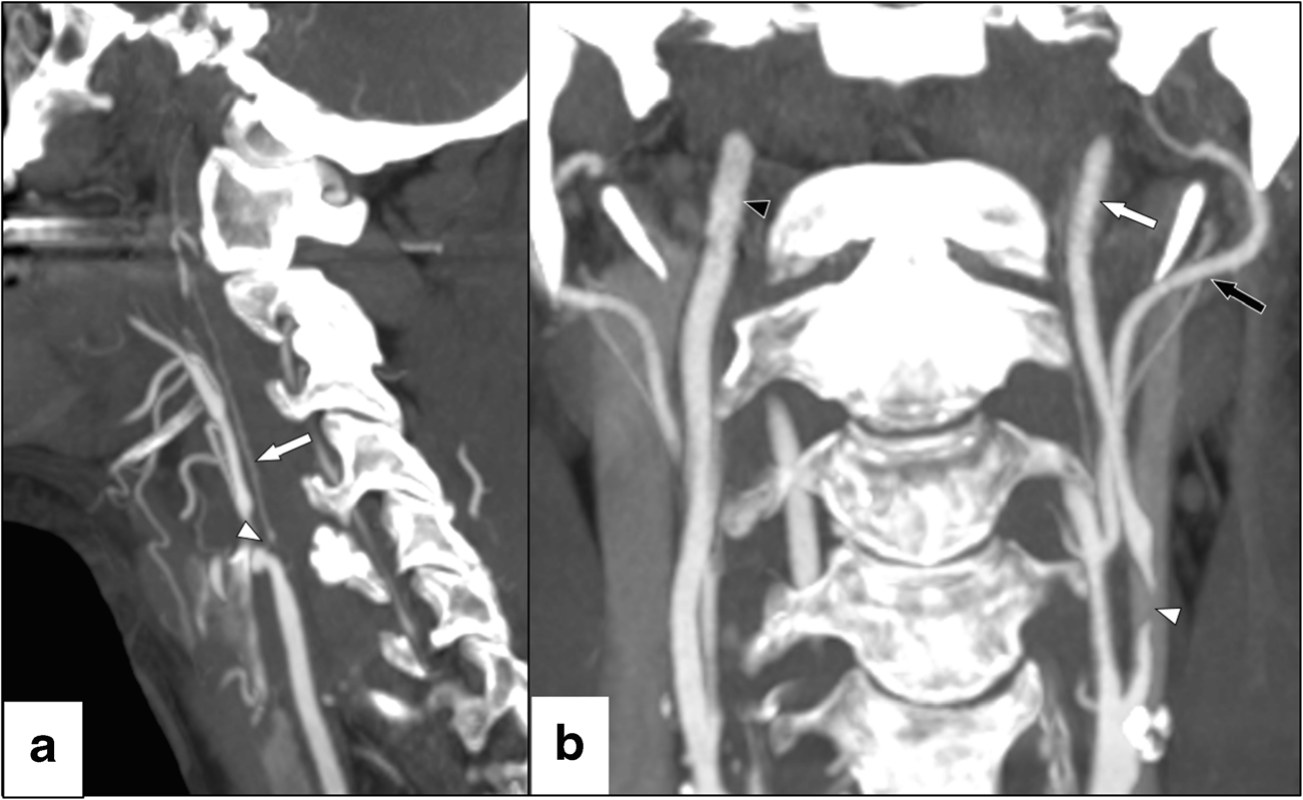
Two cases of left-sided near-occlusion. a Near-occlusion with full collapse, sagittal CTA. Beyond a severe stenosis (white arrowhead), the distal ICA is significantly size-reduced with a threadlike appearance. Distal ICA is 0.8 mm in diameter. b Near-occlusion without full collapse, coronal CTA. Beyond severe stenosis (white arrowhead), the distal ICA is normal-appearing but relatively small (white arrow, 2.8 mm diameter), smaller than contralateral distal ICA (black arrowhead, 3.9 mm diameter) and similar to ECA (black arrow, 2.4 mm diameter)
